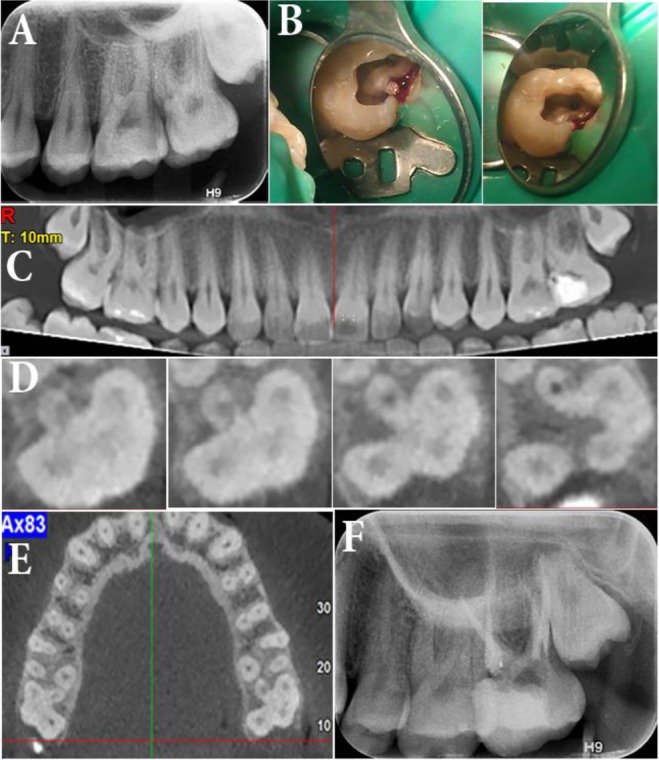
(A) Pretreatment image of the left second maxillary molar with deep cavity. Note the unusual shape of the roots. (B) Preparation of the access cavity and irregular form of the pulp chamber. (C) and (D) Cone-beam computed tomography image of the tooth which reveals the presence of four separate roots. (E) Note the second molar in the opposite quadrant which also has four roots. (F) Post-endodontic image that shows treatment of all four roots.
